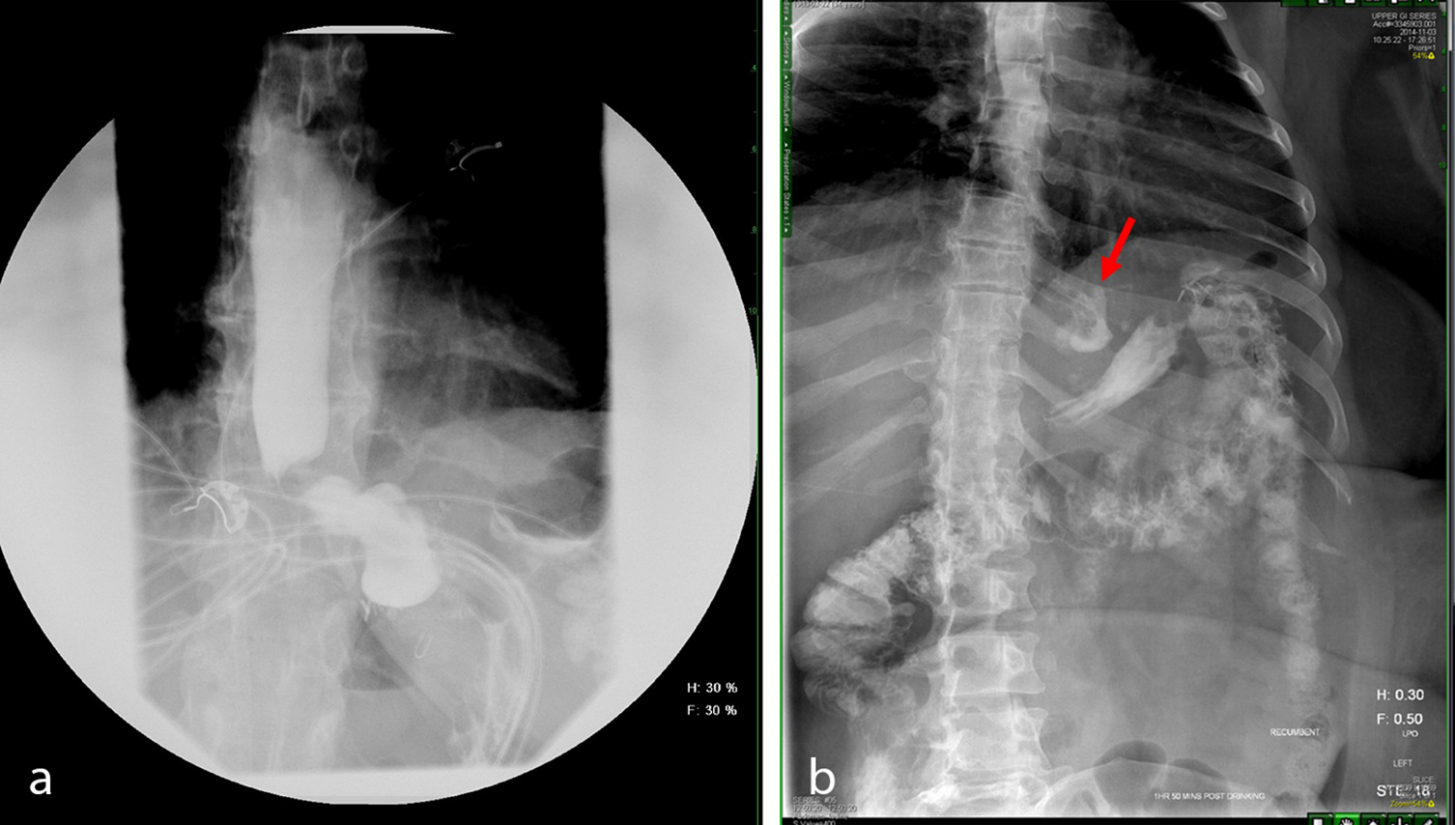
(a) Upper gastrointestinal series, early phase. (b) Upper gastrointestinal series, late phase. Arrow indicates extravasated extraluminal contrast representing leak on CT.
